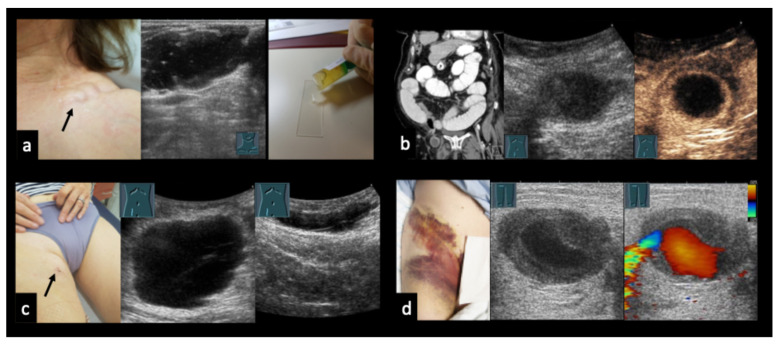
Examples of cystic-appearing, non-lymphatic masses. (a) Cystic-appearing ganglion arising from the left sternoclavicular joint; aspiration yielded clear, gelatinous fluid. (b) Right-sided incarcerated inguinal hernia on CT with a cystic-appearing inguinal structure on B-mode ultrasound. CEUS at 30 s shows absent central enhancement. (c) Right-sided postoperative mass presenting as anechoic on ultrasound; decompressive aspiration revealed serous fluid consistent with a postoperative seroma. (d) Right-sided groin mass after cardiac catheterization; B-mode ultrasound shows a hypoechoic lesion with a central anechoic area, and color Doppler confirms the diagnosis of a pseudoaneurysm. Arrow: location of the ultrasound finding.
